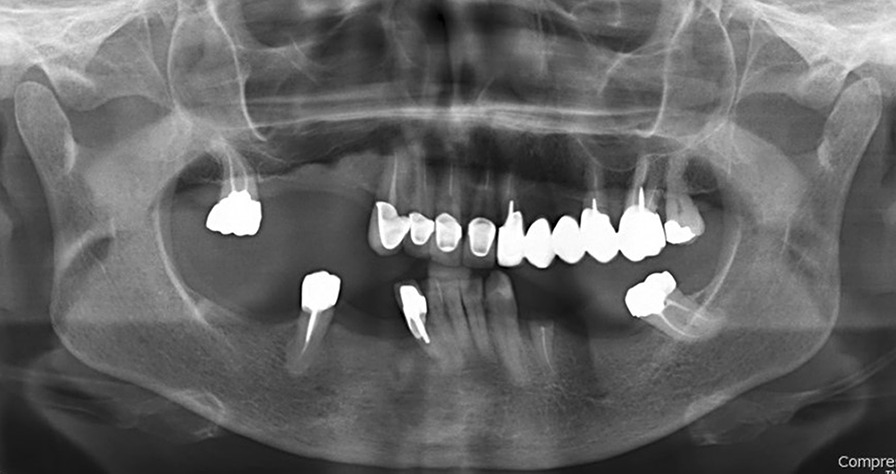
Panoramic radiography at the first consultation negative for pathological bone imaging
